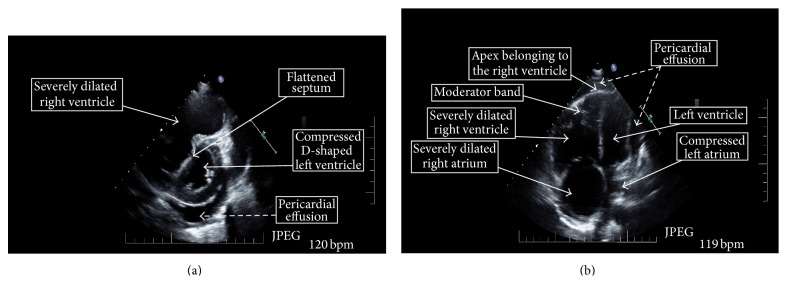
Echocardiogram of the patient demonstrating key features of severe pulmonary arterial hypertension. (a) Left parasternal short axis view. (b) Four-chamber apical view. Elevated pulmonary artery systolic pressures lead to a dilated right ventricle and right atrium. Dilation of the right ventricle causes flattening of the interventricular septum and the normally larger left ventricle becomes constricted. The rapid heart rate of 120 bpm also reduces the time for left ventricular filling and coronary artery perfusion. All of this results in hemodynamic compromise with decreased cardiac output, which can result in cardiogenic shock.
